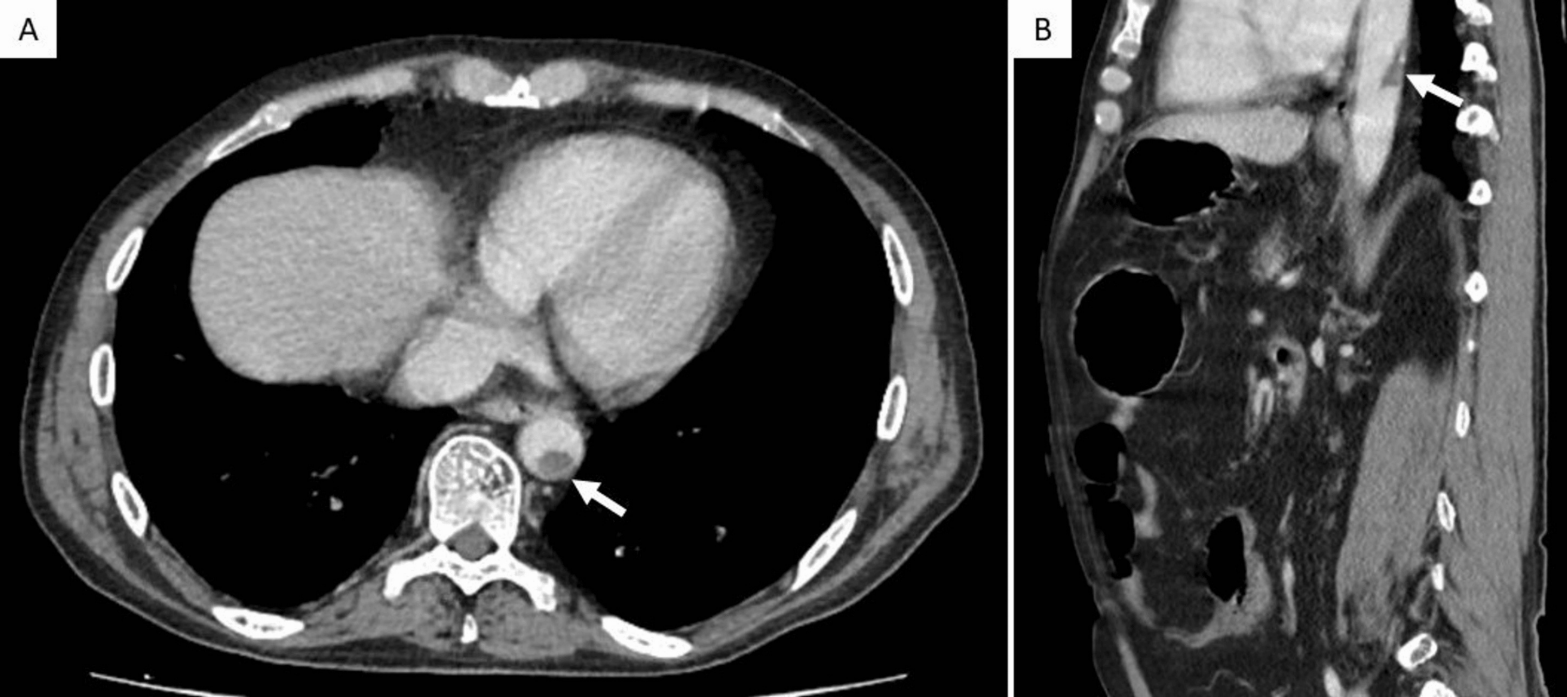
Thoracic aortic pedunculated thrombus in a computed tomography angiography performed one month prior (white arrows) Axial view (A) and sagittal view (B)
